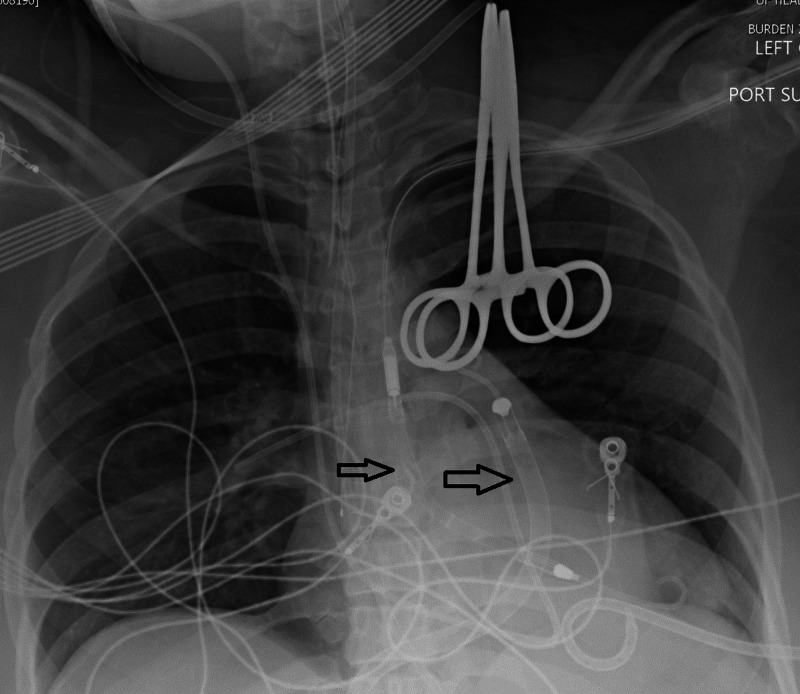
Chest X-ray showing Bi-V Impella placement. Bi-V, biventricular
